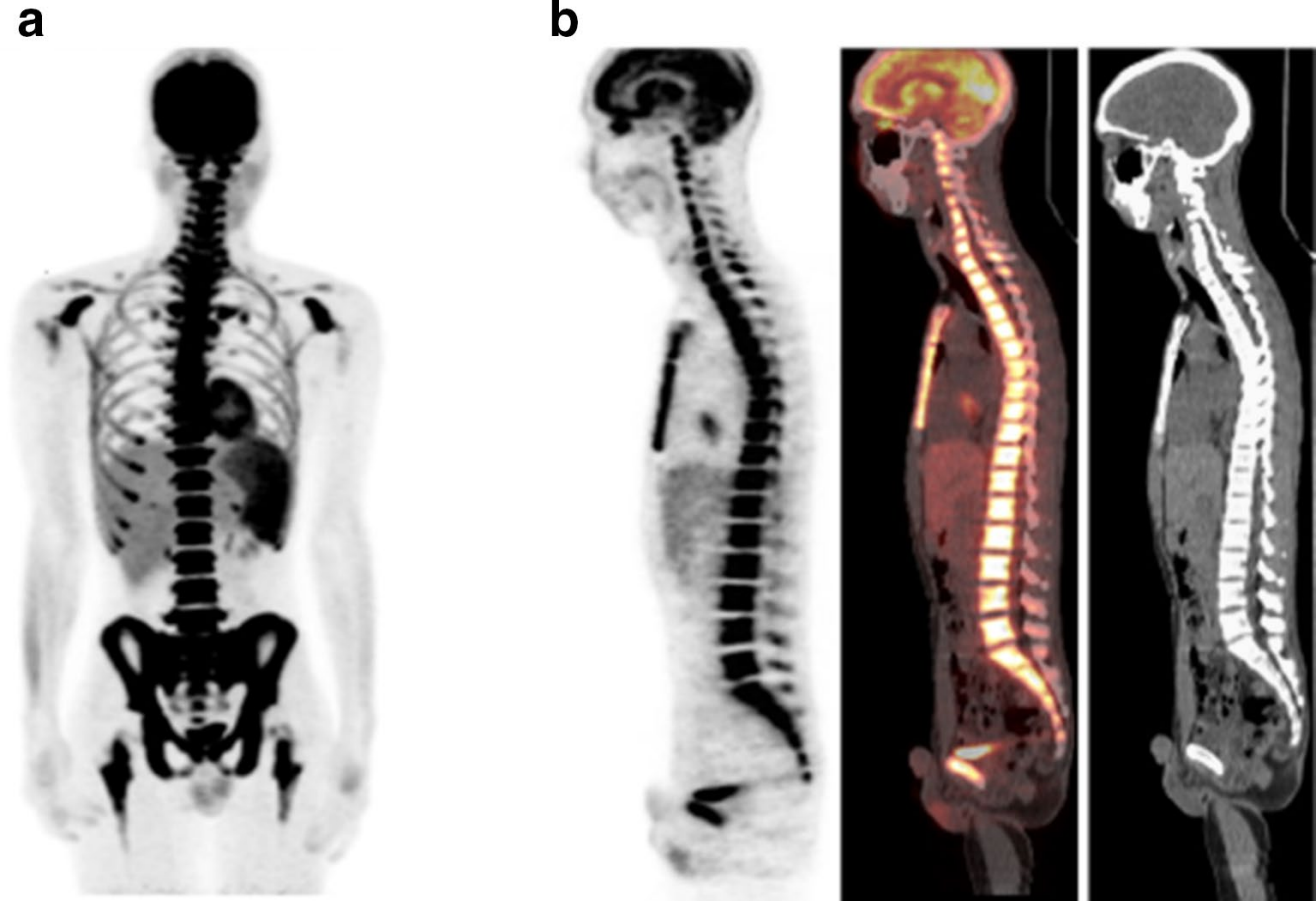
18F-FDG PET/CT images of a 24-year-old man with B-cell acute lymphoblastic leukemia (Patient 7). Maximum-intensity projection PET image (a) and sagittal PET/CT images (b), showing diffuse homogeneous bone marrow uptake in the body trunk and focal uptake in the extremities. The white blood cell count was elevated (9100/μL) and leukemia was confirmed by the detection of leukemic cells in the peripheral blood (21.5 %)
